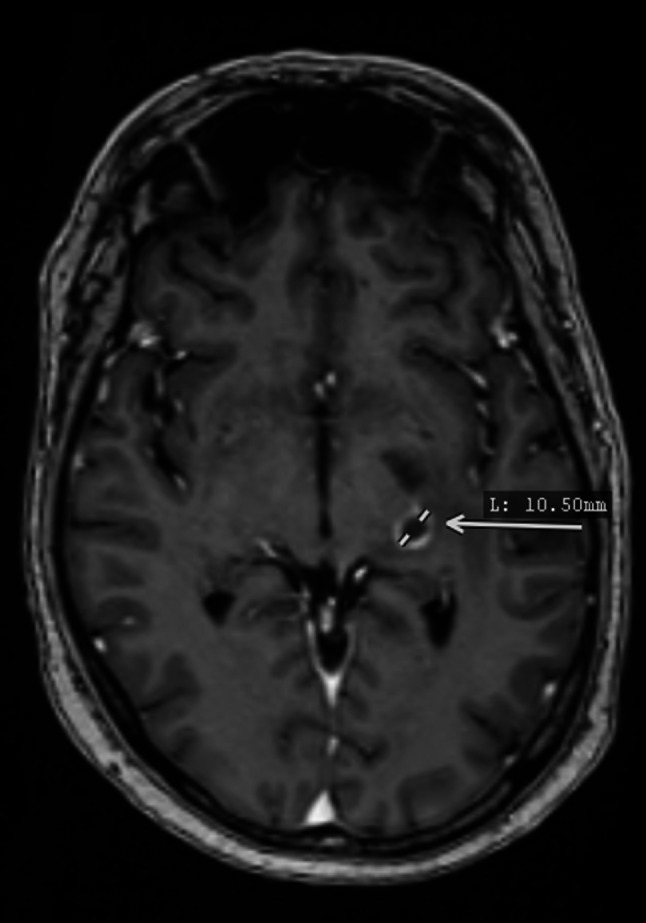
Axial contrast-enhanced T1-weighted MRI demonstrating a 10.5 mm ring-enhancing lesion in the left optic radiation, associated with mild vasogenic edema and corresponding to the patient’s visual field defect
